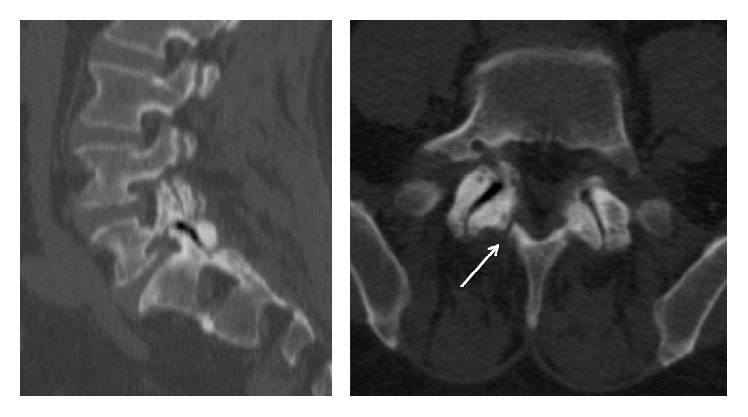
Preoperative CT. L5 interarticularis right pars spondylolysis (white arrow) and severe facet joint osteoarthritis with vacuum sign.
